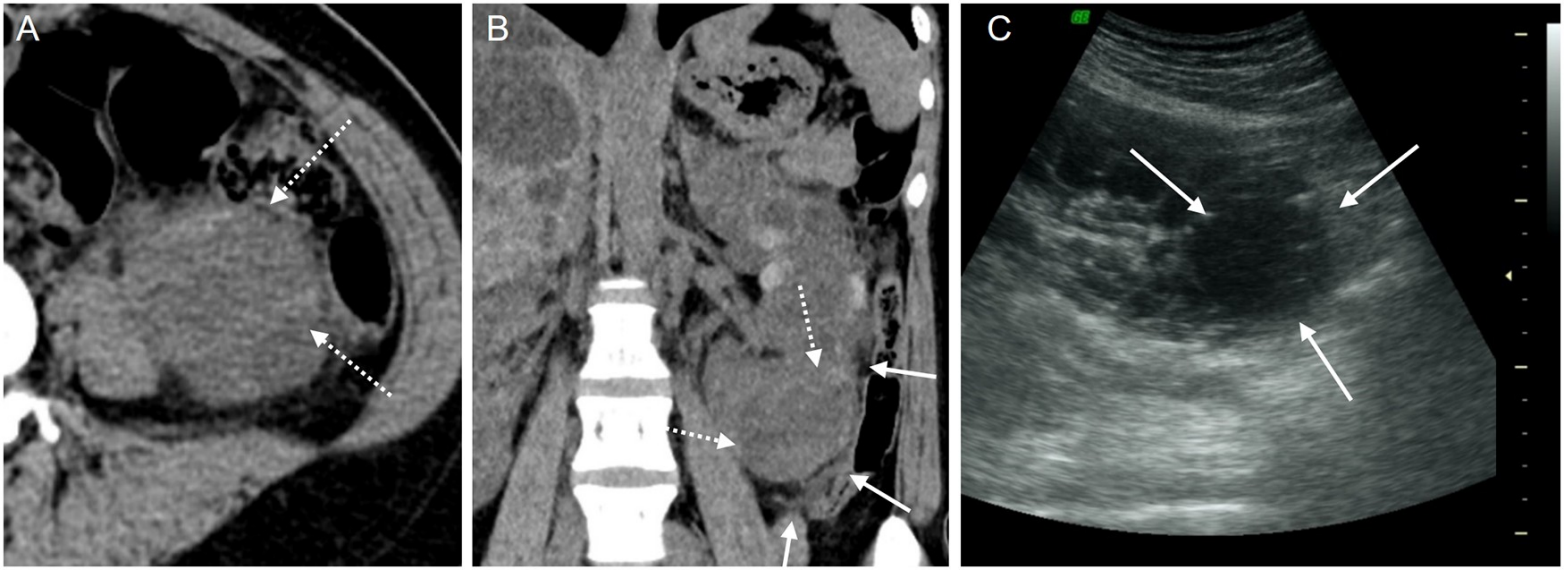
A 65-year-old female patient with an infected cyst (R10 in Fig 2). (A, B) Pre-contrast CT image shows a 4.5 cm sized cyst with a lobulated shape in left kidney lower pole (dotted arrows). The cyst has pericystic infiltration (arrows). (C) On US scan, the cyst (arrows) appears heterogeneous and echogenic.
